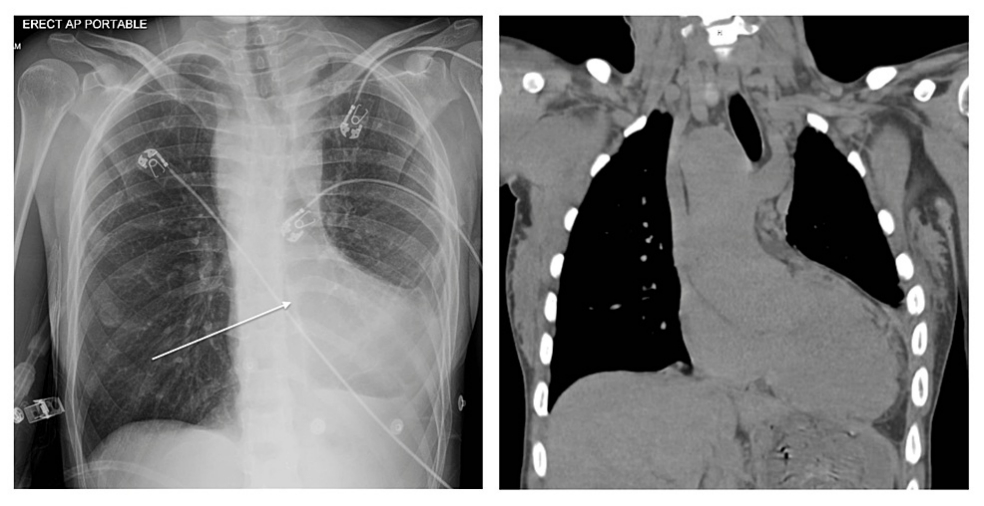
CXR and CT chest showing boot-shaped heart
